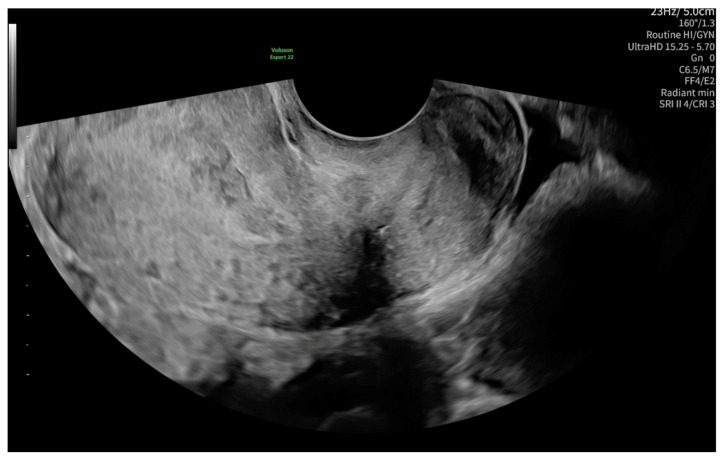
Non-specific ultrasonography image on admission, possibly suggesting the presence of cervical mucus.
